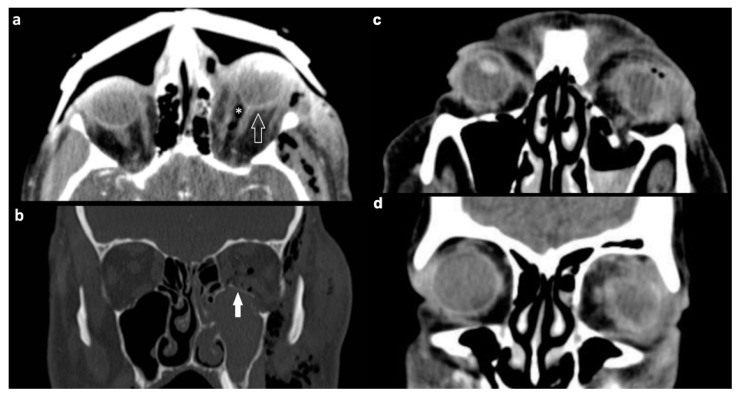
(a,b) Orbital compartment syndrome after blow-in fracture of the left orbital floor. NE-MSCT (a) Soft tissue window, axial reformation. Mass effect of a dislocated orbital floor fragment leading to left-sided exophthalmos with elongation of the optic nerve and deformation of the ocular bulb (globe tenting, indicated by hollow arrow). The posterior globe angle is reduced to 117° in the left globe, compared to 139° on the right side. Asterisk indicates intraconal air bubbles. (b) Bone kernel, coronal reformation. Solid arrow indicates the orbital floor fragment which is dislocated upwards into the orbital cavity. (c,d) Complete rupture of the left globe with irregular shape, volume loss, and air bubbles in the anterior chamber. NE-MSCT, soft tissue window in (a) Axial and (b) coronal reformation (NE-MSCT = non-contrast-enhanced multi-slice CT).
